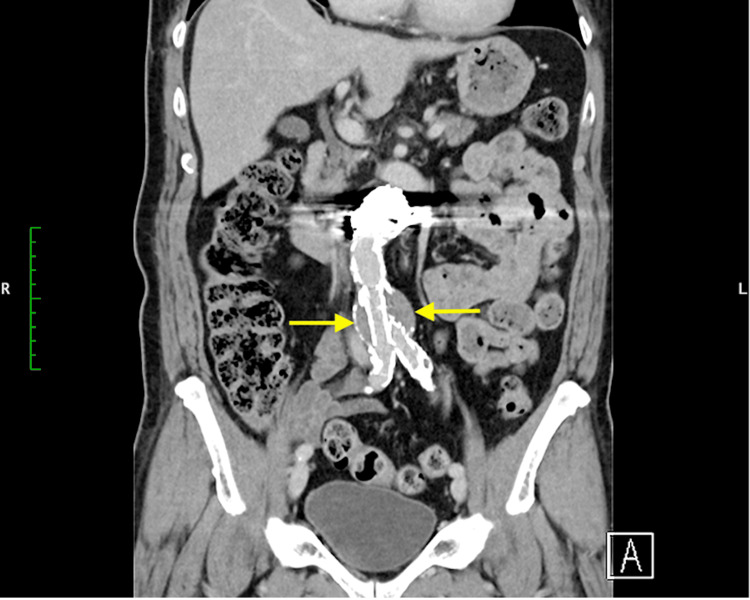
As observed in the CT image, it is possible to measure the maximum short diameter of the aneurysm as the coil is placed only in the neck.
